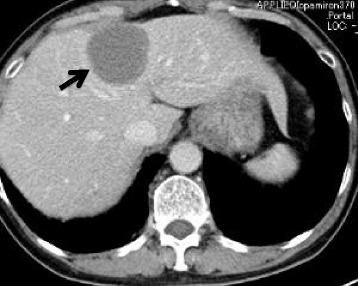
Enhanced computed tomography revealed a solitary, low-density, and hypovascular tumor with a diameter of 54 mm in segment 4 of the liver (*arrow*)
